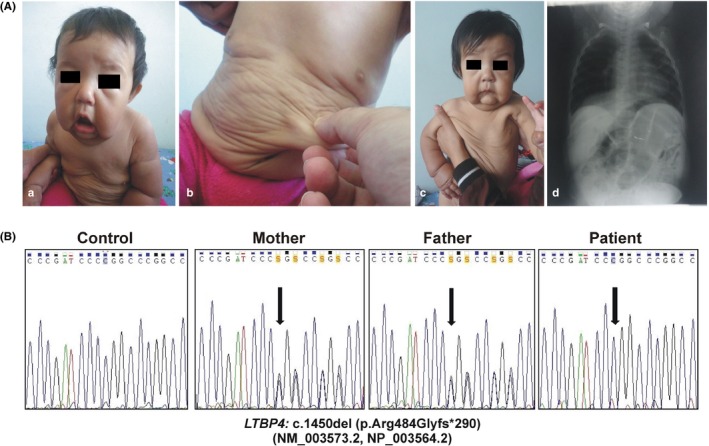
(A) Clinical appearance of the patient. At 9 months of age (a, b), a diagnosis of CL was given for the presence of loose, wrinkled, sagging, and redundant skin, and several craniofacial features. On examination at 13 months of age (c), normocephaly (between 25th and 50th percentile), dysmorphisms, that is, narrow forehead, down slanting palpebral fissures, periorbital fullness, epicanthus, hypertelorism, long philtrum, fat midface, depressed nasal bridge, anteverted nares, micro‐retrognathia, and short neck were observed. Cutis laxa was evident on cheeks (with a prematurely aged appearance), neck, axillae, arms, abdomen, glutei, and limbs. Thorax and abdomen radiography, performed at 10 months of age (d), showed discreetly prominent aortic arch with mild tortuosity, diaphragmatic eventration, normal pulmonary parenchyma with atelectasis in the left lung, and elongated gastrointestinal tract with dilatation and tortuosity. (B) Molecular analysis. Sequence chromatograms showing the position of the c.1450del (p.Arg484Glyfs*290) variant (arrows) identified in the patient in homozygosity in exon 12 of the *LTBP4* gene. Both healthy parents were heterozygous carriers. Mutation is annotated according to HGVS nomenclature (http://www.hgvs.org/mutnomen; NM_003573.2, NP_003564.2)
